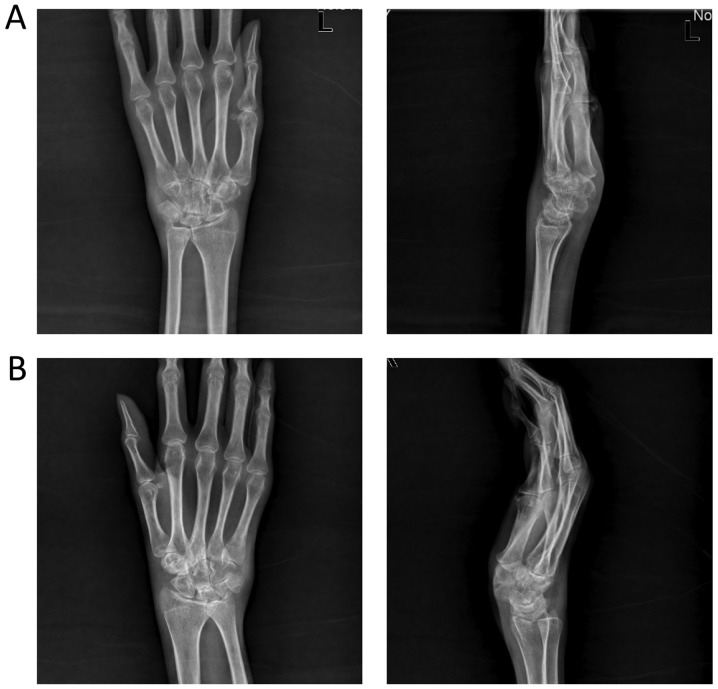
Joint X-rays of (A) left and (B) right wrists.
